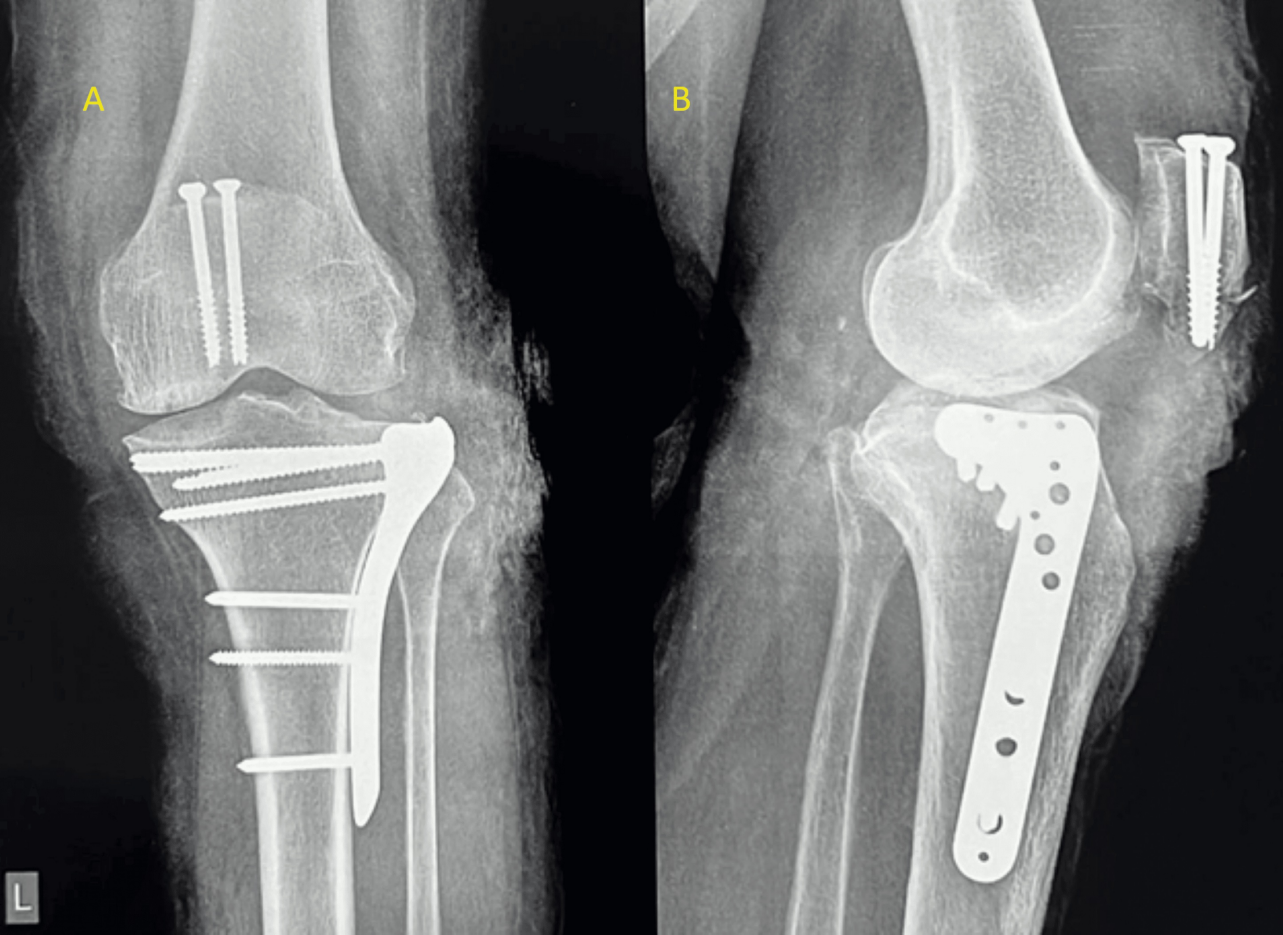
Postoperative radiograph of the left knee Radiograph showing a cannulated compression screw fixation for the inferior pole of the patella and buttress plating for the tibial plateau fracture (A) Anteroposterior view; (B) lateral view
